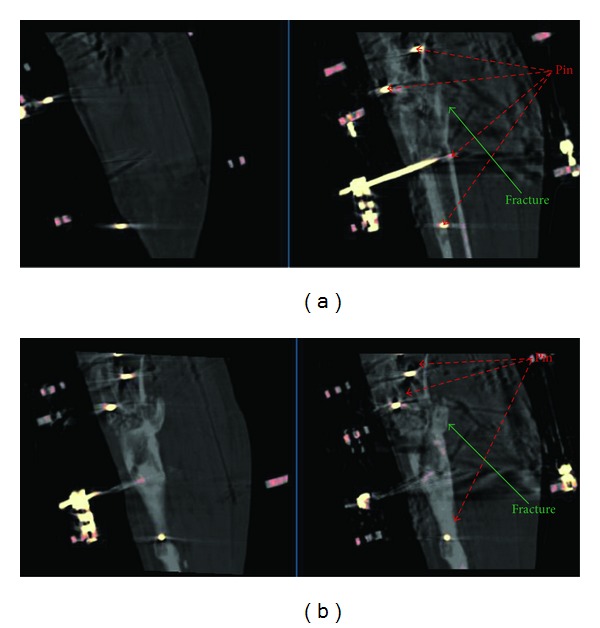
A sagittal CT section for Patient 1 showing the original CT misalignment between the first CT at 40 days (right) and the second CT at 84 days (left) after the operation to attach the TSF (a). A sagittal CT section for Patient 1 showing the final CT transformed alignment between the first CT at 40 days (right) and the second CT at 84 days (left) after the operation to attach the TSF (b).
